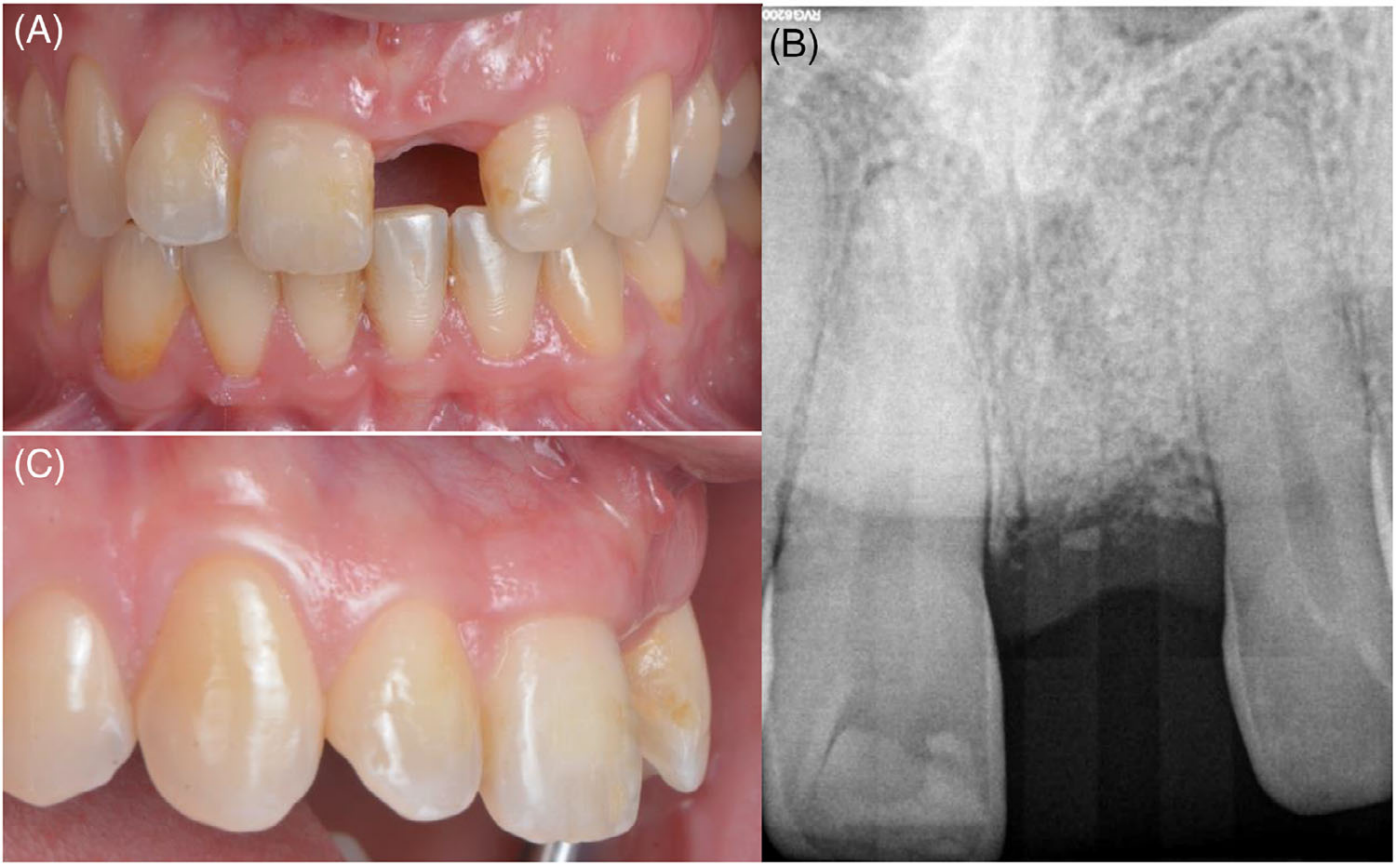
Healing at 4 months. Frontal view (A). Lateral view (C). Periapical radiograph (B).
